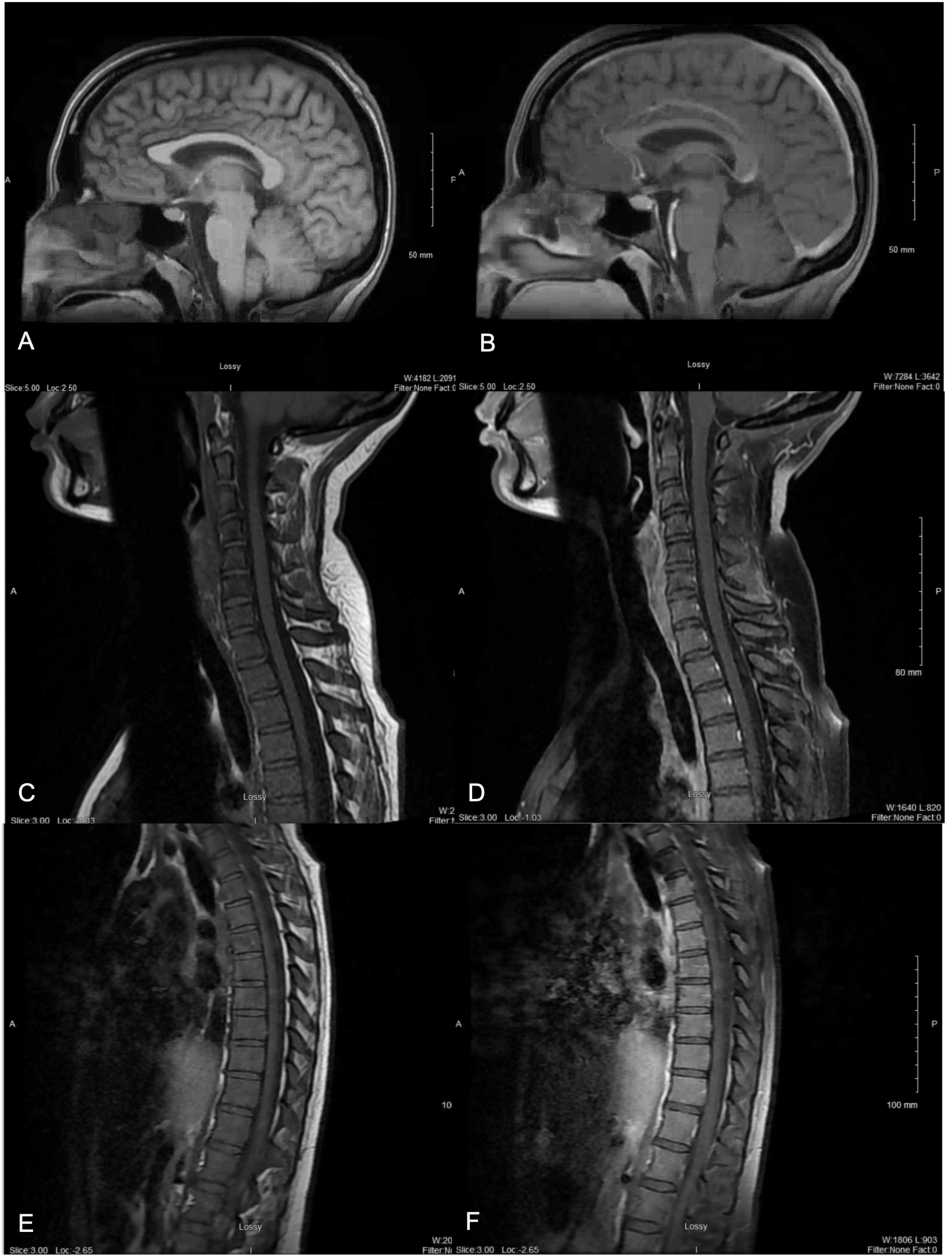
Initial neuraxial MRI with and without contrast, sagittal view. MRI taken at symptom onset. No acute findings noted. (A) Brain MRI without contrast; (B) brain MRI with contrast; (C) cervical MRI without contrast; (D) cervical MRI with contrast; (E) thoracic MRI without contrast; (F) thoracic MRI with contrast.
